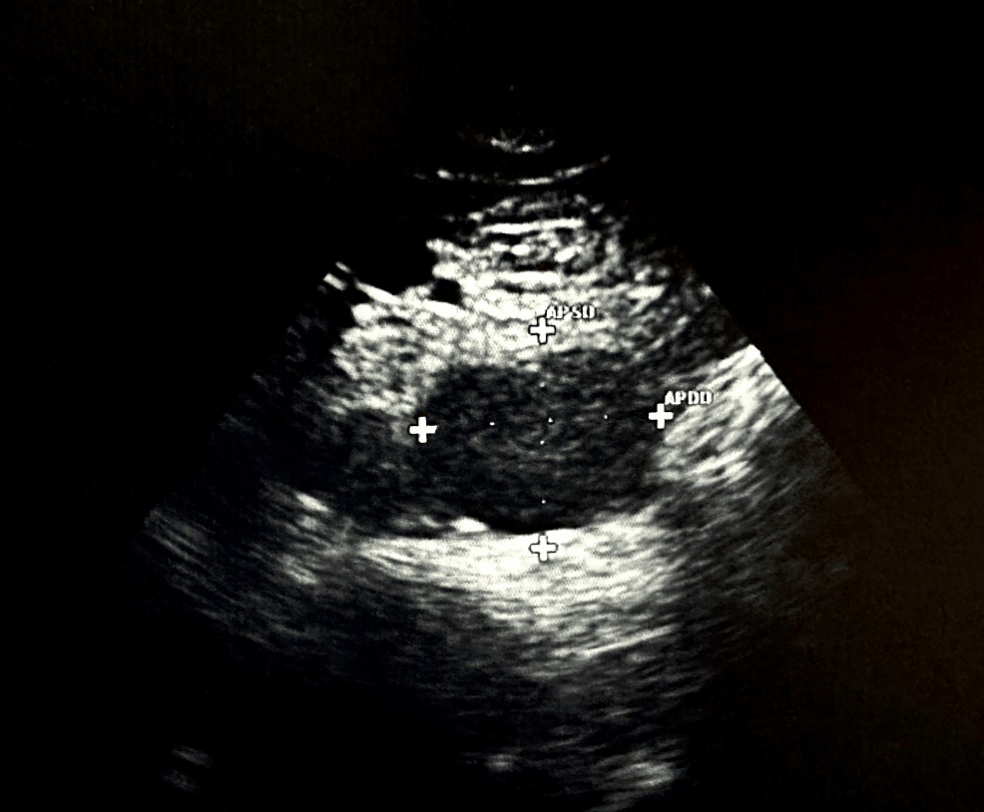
Abdominal ultrasound demonstrating the suprarenal aortic aneurysm measuring 3.8 cm in the proximal aorta.
